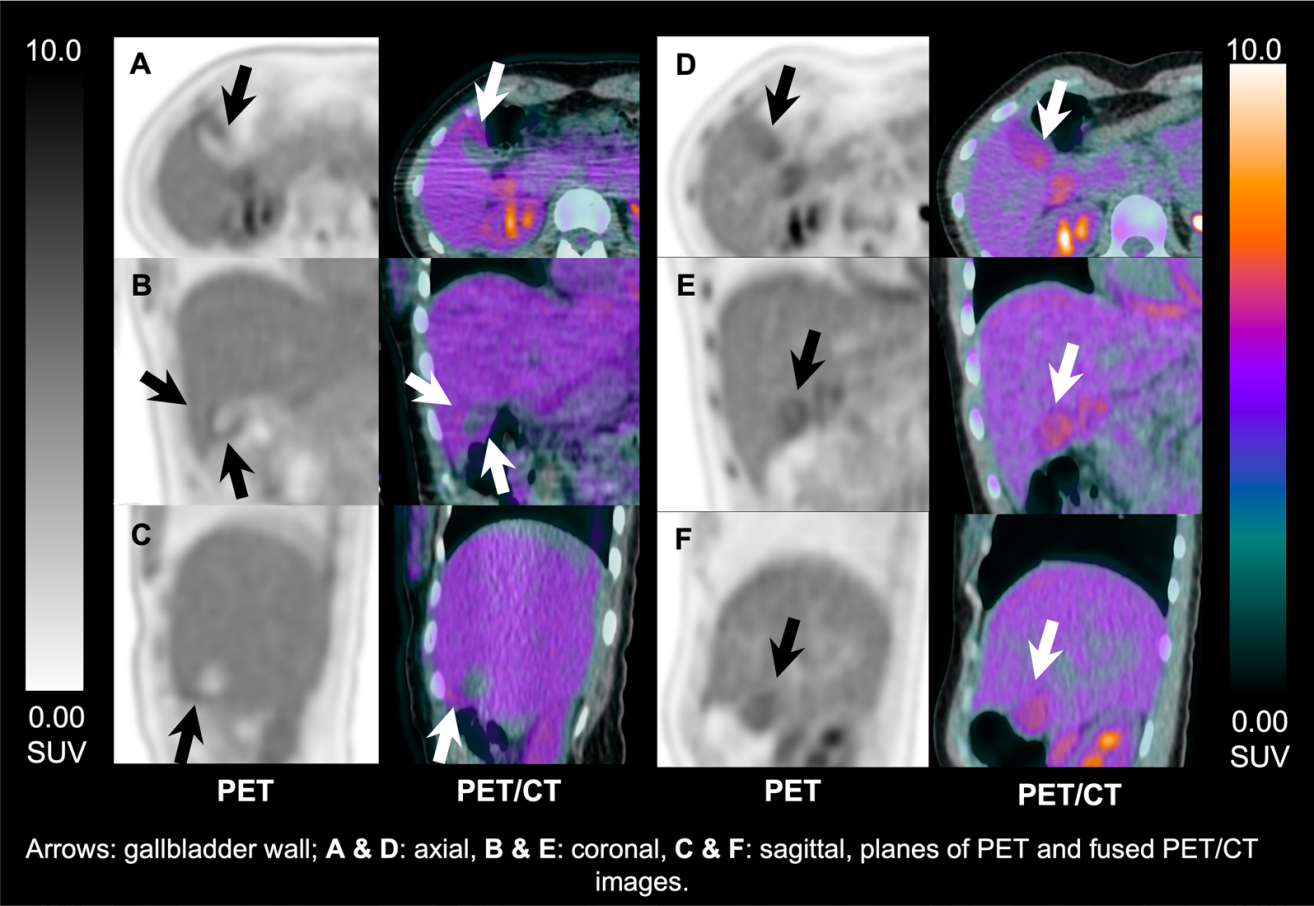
^18^F-FDG uptake patterns in the gallbladder wall and lumen. PET and fused PET/CT images after i.v. injection of 379 MBq in a 30 y/o healthy participant. A–C 40 min post injection scan shows gallbladder wall activity, equal to the liver; D–F 180 min post injection scan shows gallbladder luminal activity, higher than the liver
